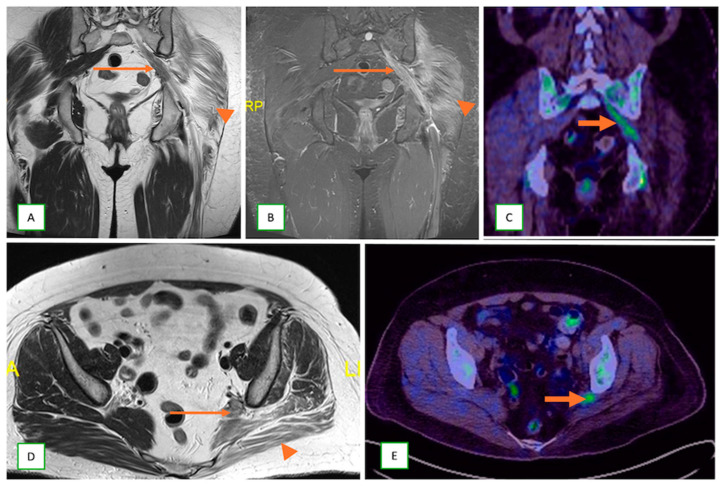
Endometrial carcinoma in a 35-year-old woman. Coronal T1 (A), coronal fat-saturated T2 (B), coronal fused PET-CT (C), axial T2 (D), and axial fused PET-CT (E) images showing endometriotic deposits in the left sciatic nerve (thin arrows) with FDG-avid activity demonstrated on FDG-PET fused images (thick arrows). Denervation edema and fatty atrophy are also seen in the left-sided gluteal muscles (arrowheads).
